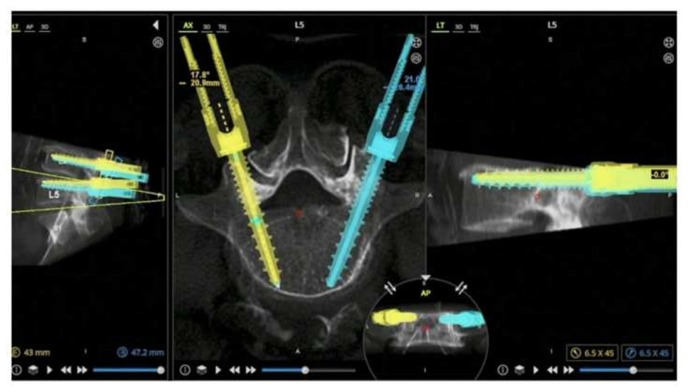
Preop CT planning of the pedicle screws in axial and sagittal view.
